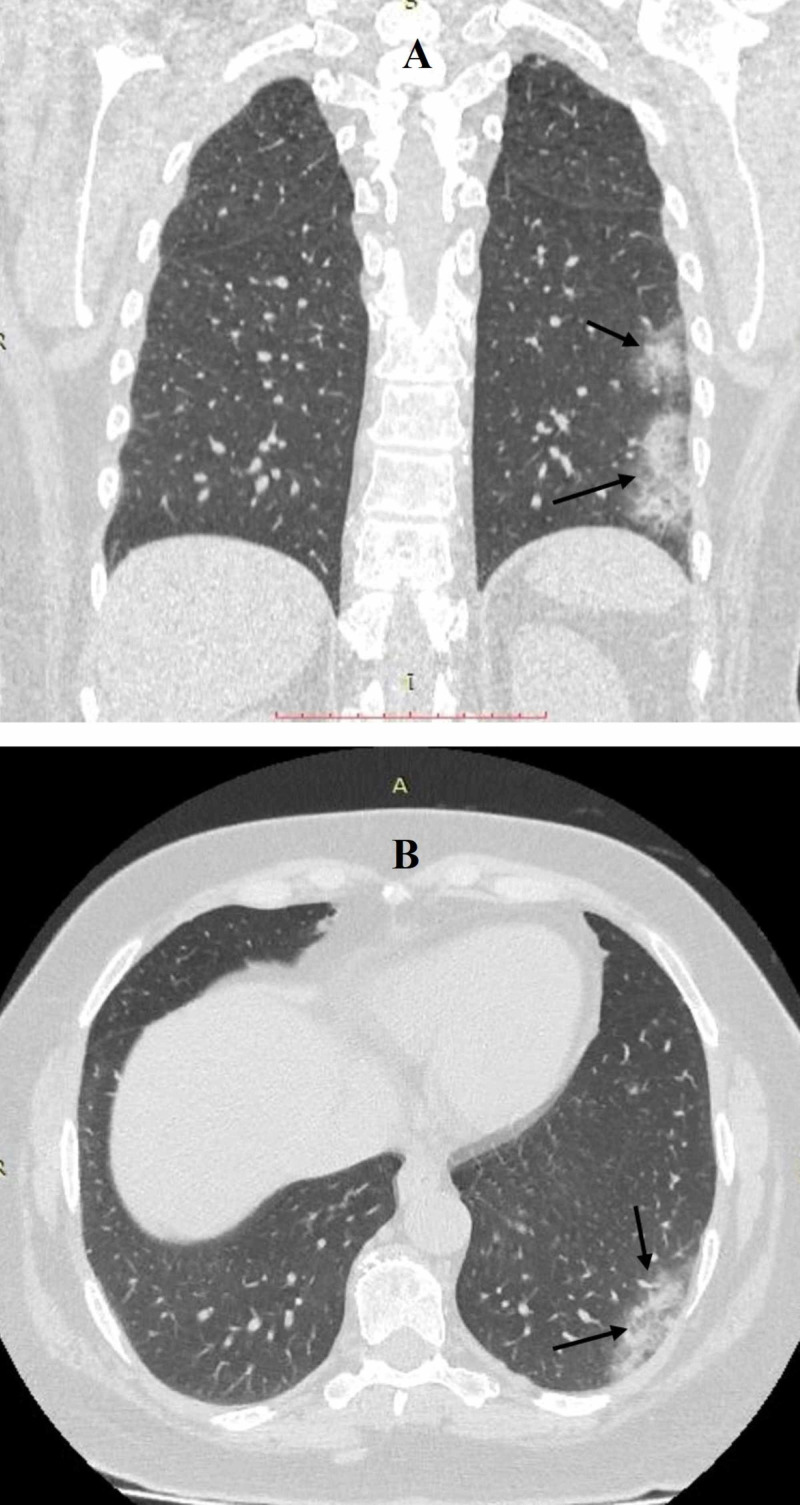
Coronal (A) and axial (B) sections of CT chest showing infiltrates in the apico-posterior and posterior basal segments of the left lung.
